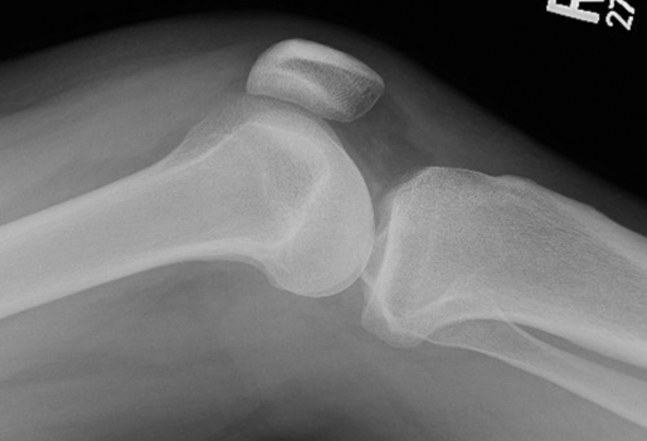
Lateral knee x-ray showing joint effusion with no visualized foreign body.
